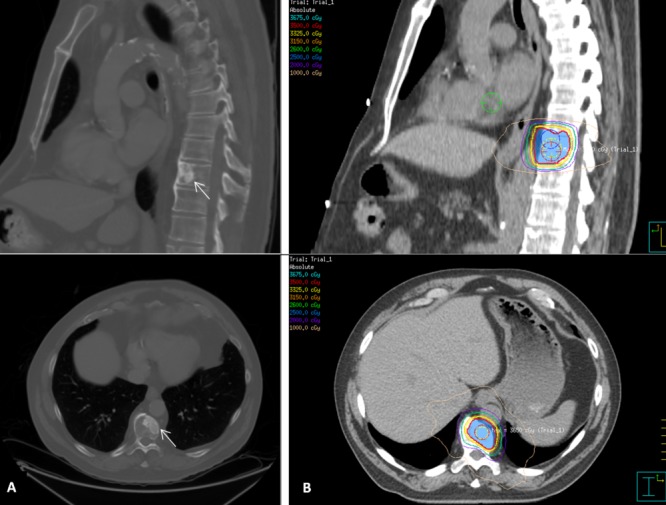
A) Sagittal and axial CT slices showing the T10 oligometastasis. B) Stereotactic body radiotherapy treatment plan and dose distribution.
